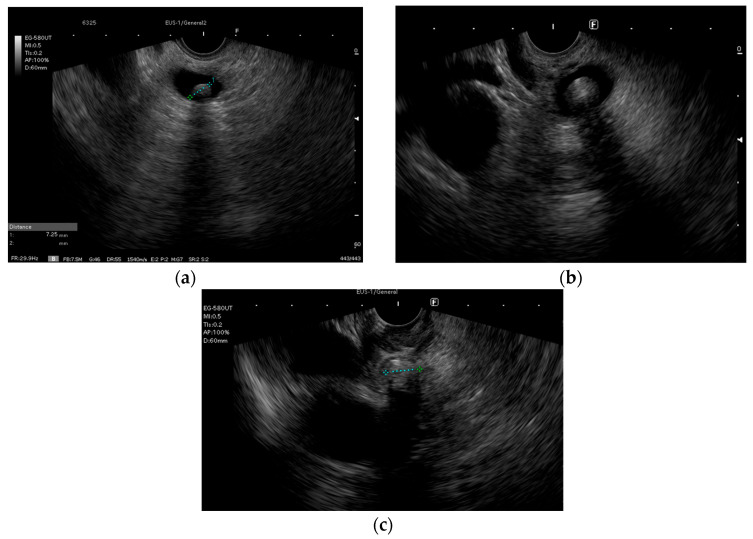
(a) Endoscopic ultrasound diagnostic of choledocholithiasis; (b) infracentimetric stones in the common bile duct; (c) stone impacted near the papilla in the common bile duct.
